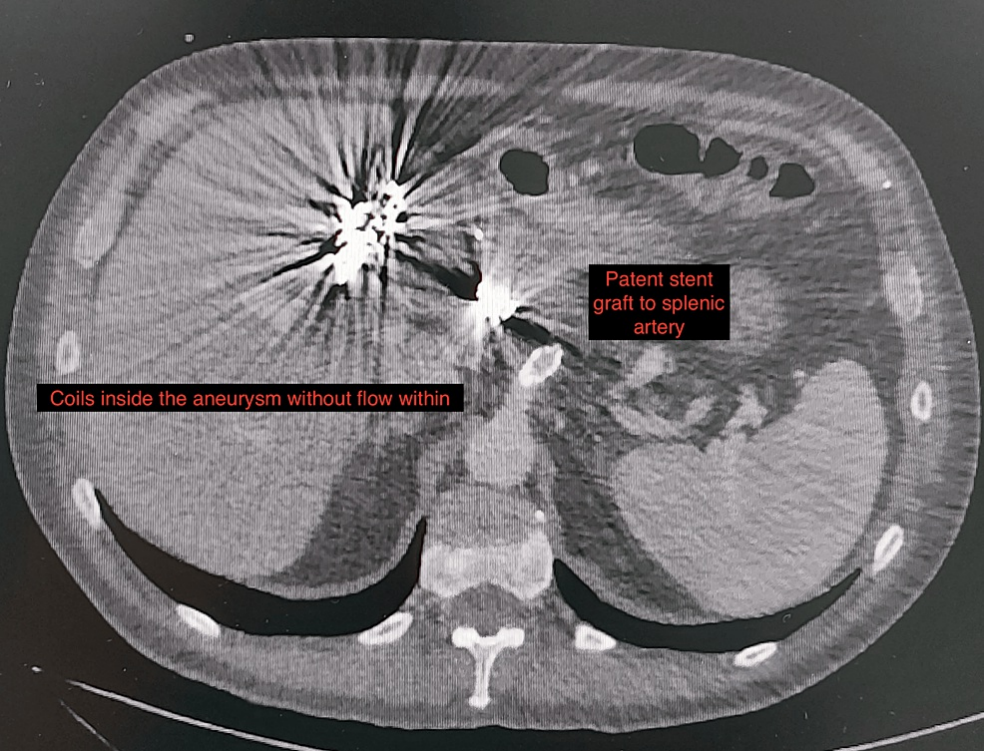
CTA at six months showed shrinkage of the aneurysm with no flow within and patent stent to the splenic artery. CTA: computed tomography angiography
